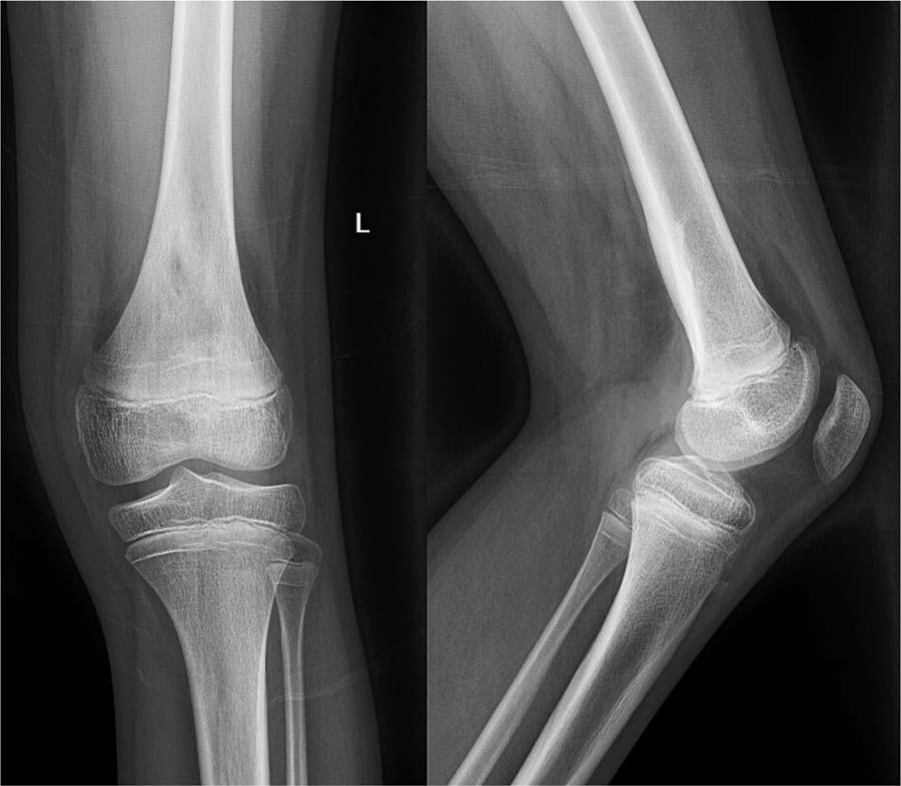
Radiography obtained 1-year postoperatively, demonstrating no acute osseous changes.
